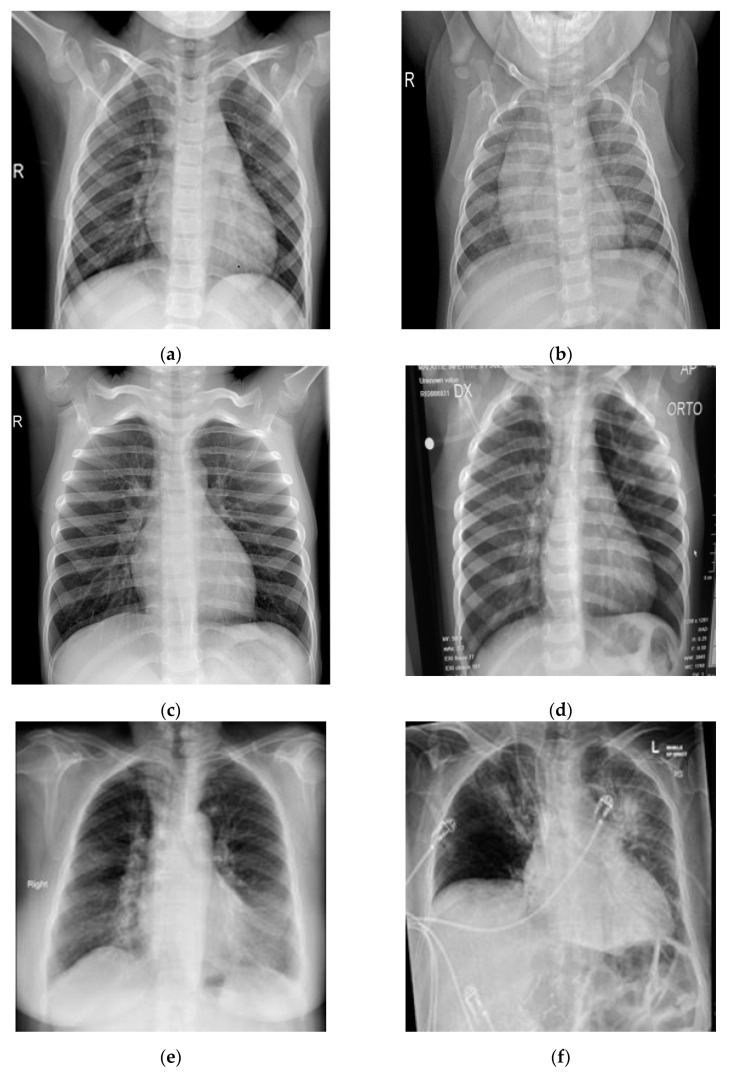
Samples of chest X-ray images assessed via Kaggle database [30,31,32] for training purposes: (a–c) normal chest X-rays; (d–f) COVID-19 chest X-rays.
